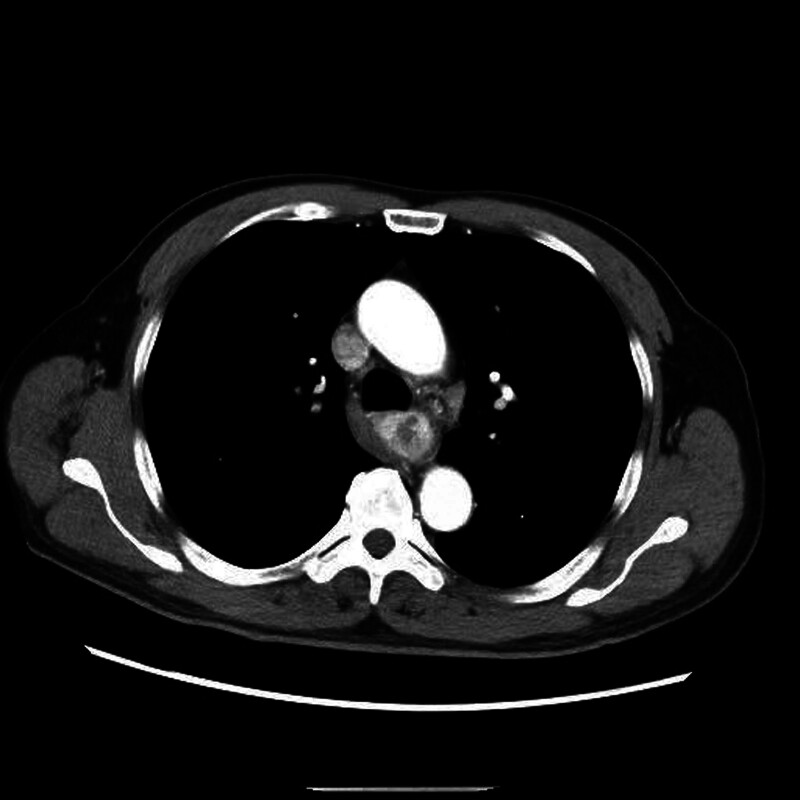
CT scan showing the mid-thoracic esophageal mass. CT = computed tomography.
